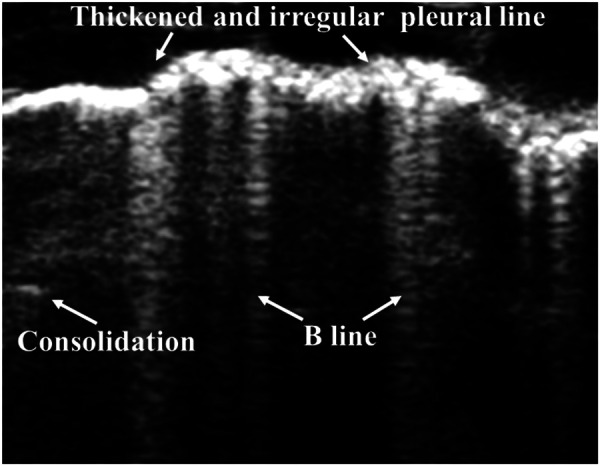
Bronchopulmonary dysplasia on lung ultrasonography. Ultrasound shows unequally distributed B-lines, thickened pleural lines, and multiple different-sized subpleural consolidations.
